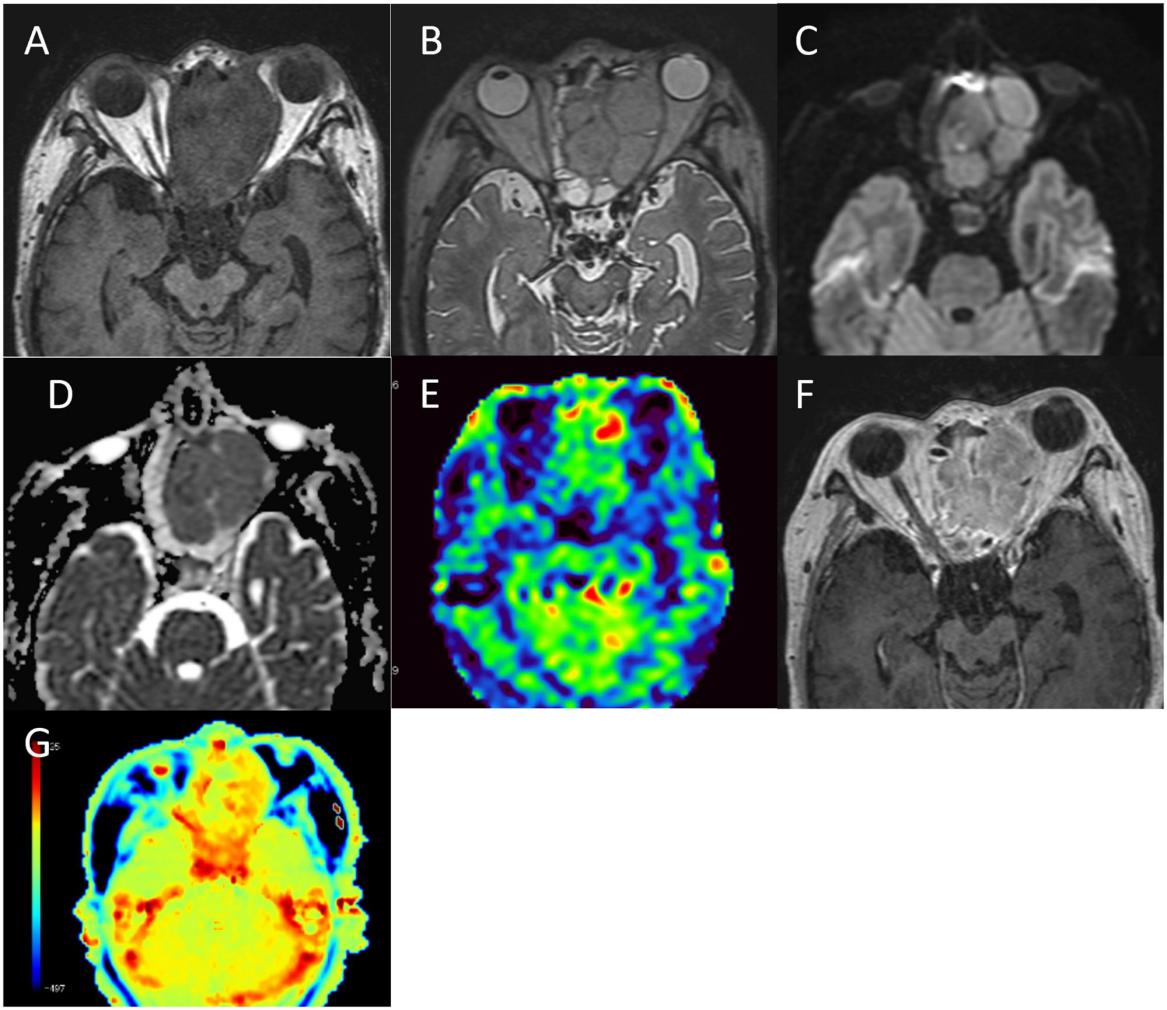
(A-G) MRI three months after admission. MRI shows a recurrent tumour in the left ethmoid sinus with an isointense signal in comparison to muscle on T1WI (A), hyperintense signal on T2WI (B) and a hyperintense signal with a low ADC value (mean ADC value 0.91 × 10^−3^ mm^2^/s) on DWI (C, D). On ASL-PWI, the signal of the tumour is isointense in comparison to normal brain parenchyma (E) and Gd-T1WI shows homogeneous enhancement (F). APT-CEST imaging shows a hyperintense signal in the tumour in comparison to the normal brain parenchyma (G).
